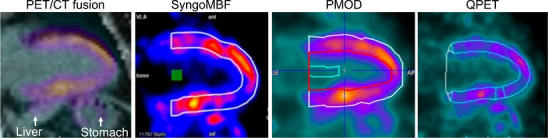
Representative images demonstrate that liver spillover affects automated placement of myocardial VOIs in the inferior wall. The leftmost panel shows a fused PET/CT image, with the liver and stomach indicated by white arrows. Automated VOIs include varying degrees of adjacent tissue. Therefore, manual correction is required for accurate myocardial delineation.
